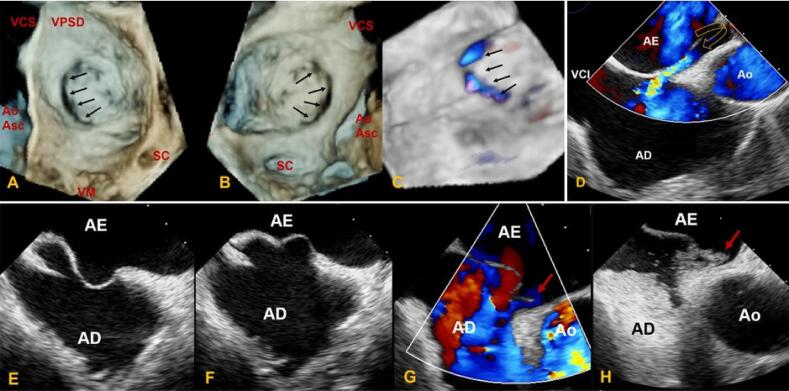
Visão en face ecocardiográfica transesofágica tridimensional (modo 3D Zoom) do SIA, mostrando o aneurisma da lâmina da FO associado a descontinuidade elíptica (setas) entre os átrios de perspectiva atrial esquerda (A) e direita (B) e confirmada com Doppler em cores (C). (D) Imagem transesofágica bidimensional de FOP ‘‘stretched “ (seta curva) em visão bicaval com fluxo ao Doppler colorido da esquerda para a direita. (E, F) Imagem transesofágica bidimensional do SIA com mobilidade excessiva da lâmina da FO e (G) FOP associado (seta vermelha) demonstrado ao Doppler colorido com fluxo da esquerda para direita e passagem intermitente de microbolhas de salina agitada da direita para esquerda (H). AD: átrio direito; AE: átrio esquerdo; Ao: aorta; Ao Asc: aorta ascendente; FO: fossa oval; SC: seio coronariano; SAI: septo interatrial; VCS: veia cava superior; VCI: veia cava inferior; VM: valva mitral; VPSD: veia pulmonar superior direita.
